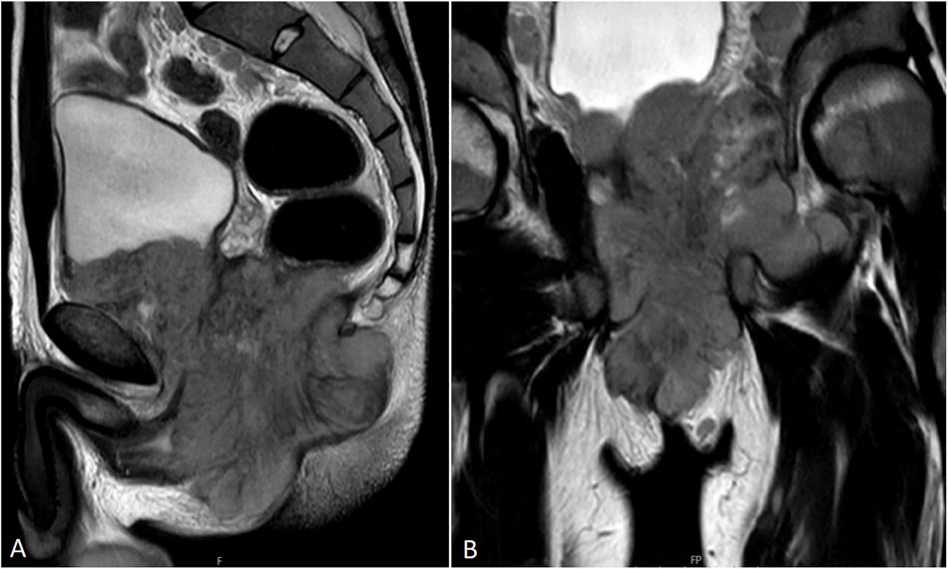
Selected sagittal (A) and coronal (B) T2W Pelvic MRI images better demonstrate the extension of the mass, which is noted to invade the UB base superiorly. Multiple enlarged pelvic lymph nodes are also seen.
